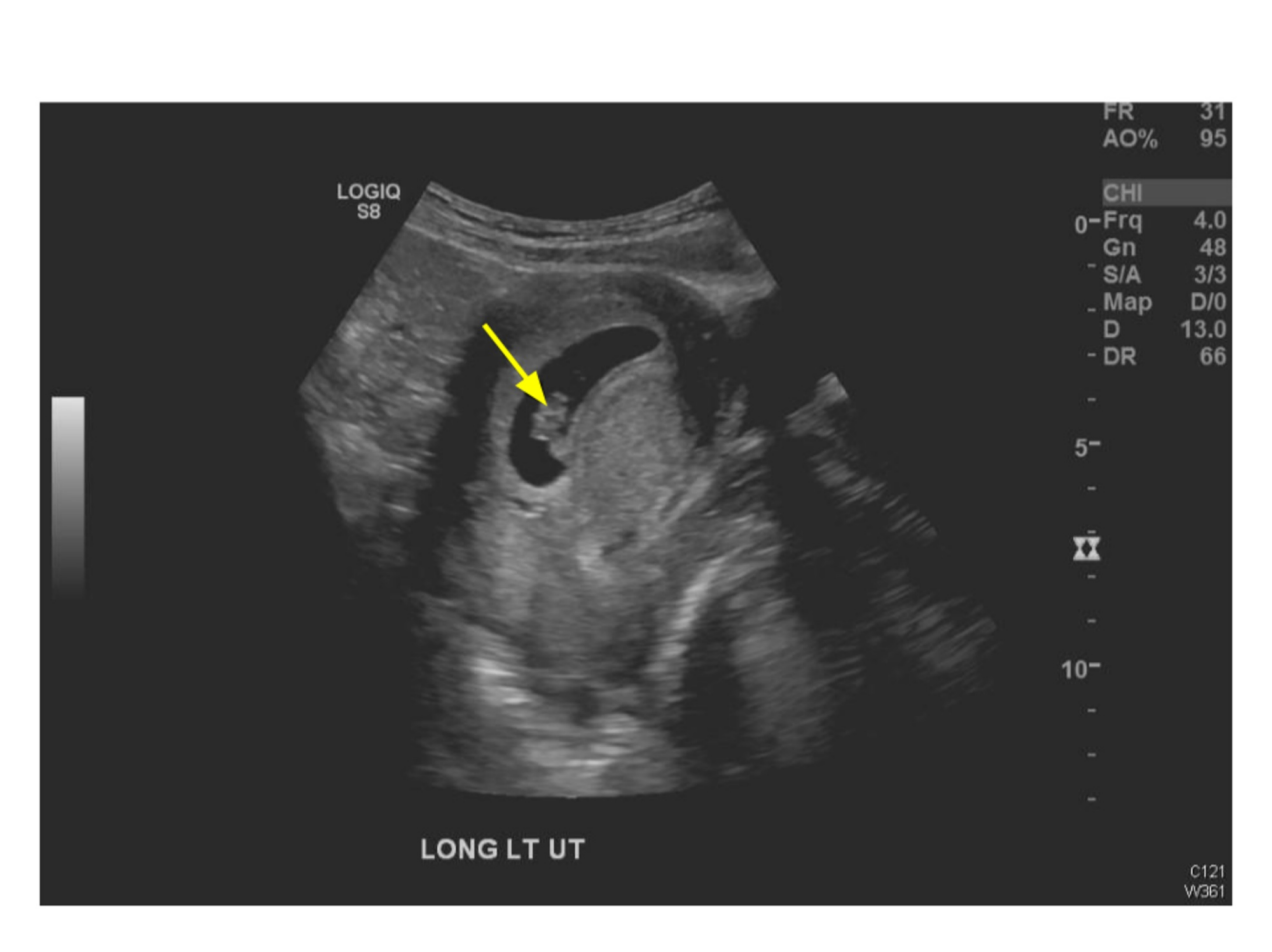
Sonogram demonstrating an intrauterine pregnancy.
